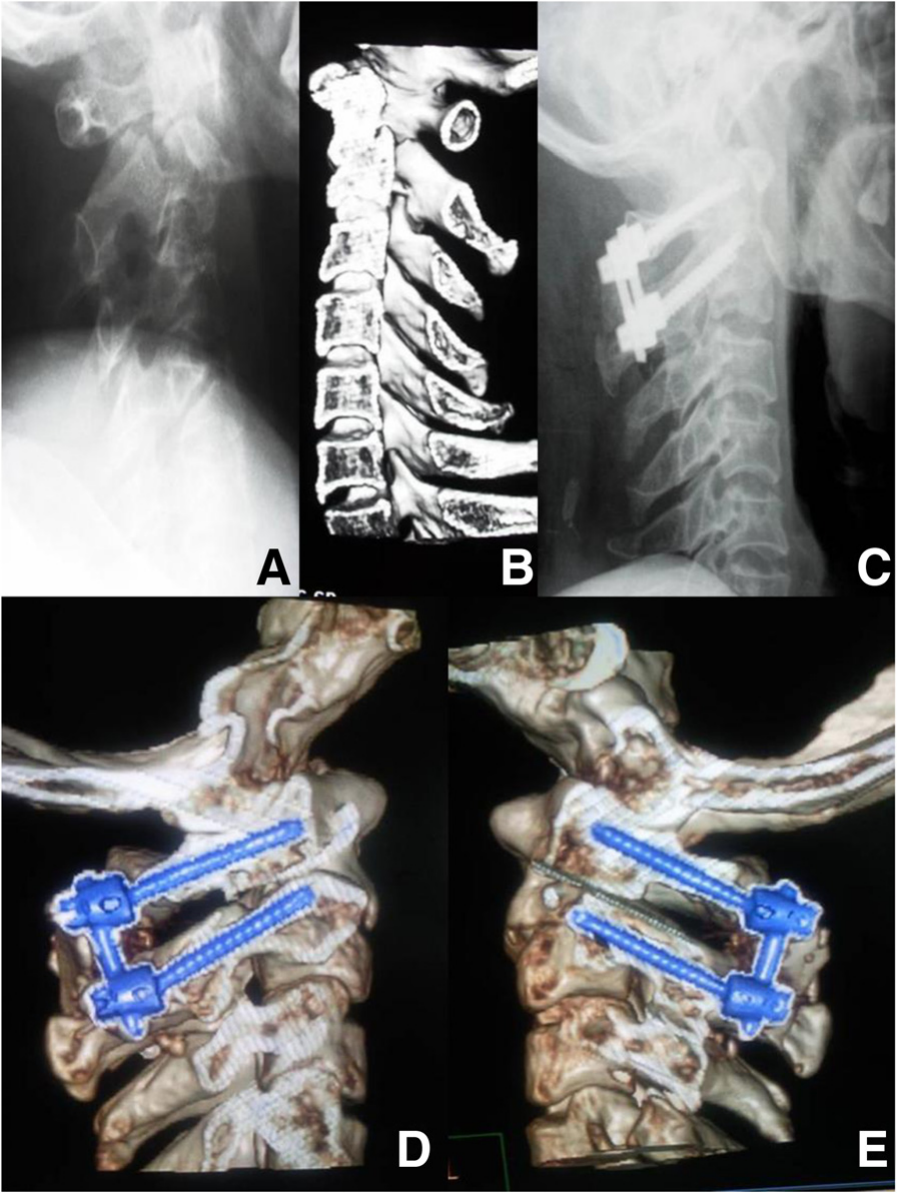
A patient who presented with an odontoid fracture underwent atlantoaxial fusion and internal fixation. a and b Lateral X-ray and sagittal reconstruction CT scans showing odontoid fracture. c Lateral X-ray after surgery revealing good fracture reduction and internal fixation. d and e Postoperative sagittal reconstruction CT scans showing atlantoaxial internal fixation with atlas pedicle screw and axis pedicle screw
